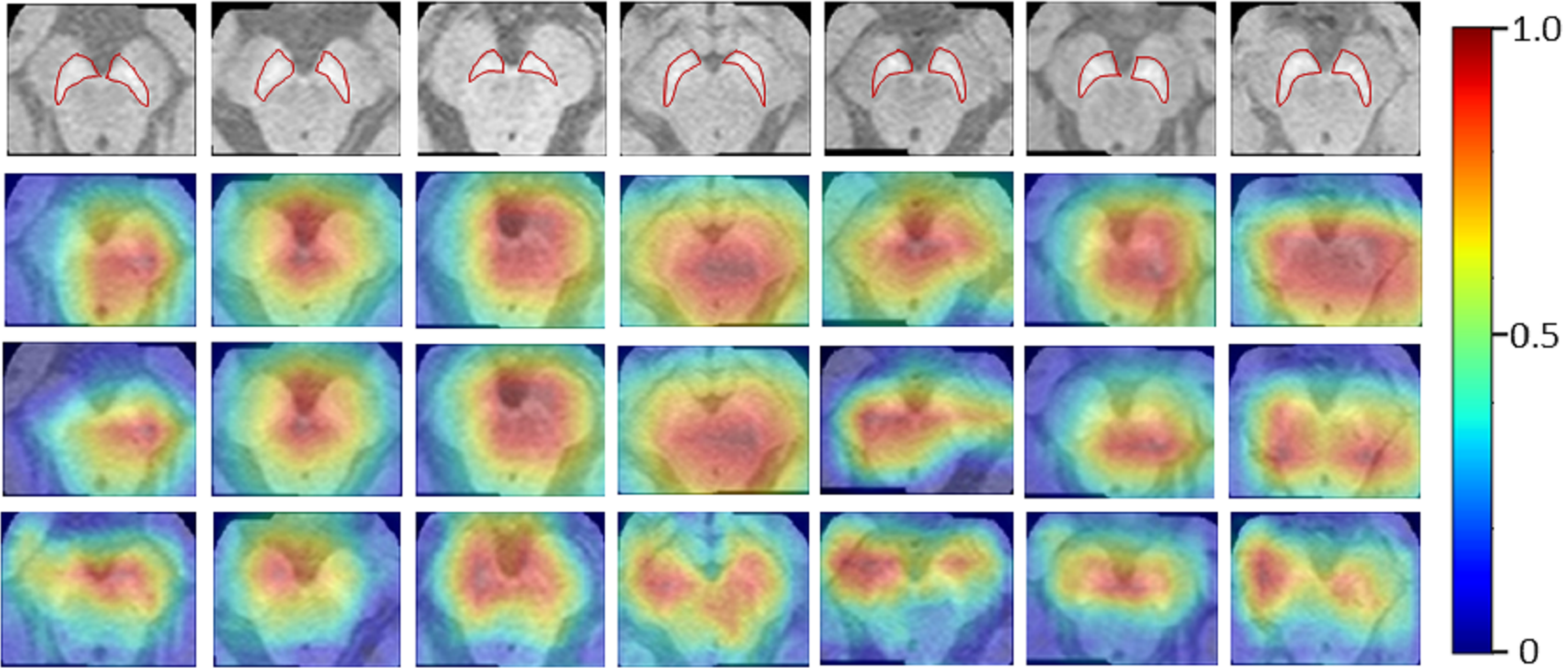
Results for the Neuromelanin MRI-PD dataset. It can be clearly observed that HR-CAMs (row 4) perform better compared to the other two CAM methods (row 2 (Zhou) and 3 (GradCAM)) resulting in activations for the left and the right SNc separately.
